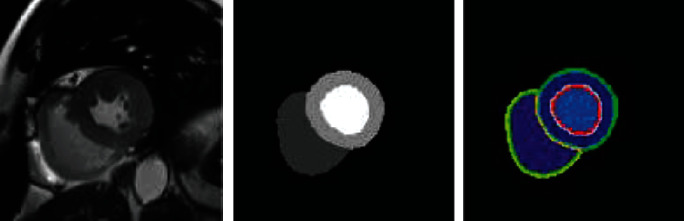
Example of MRI from ACDC challenge dataset.
